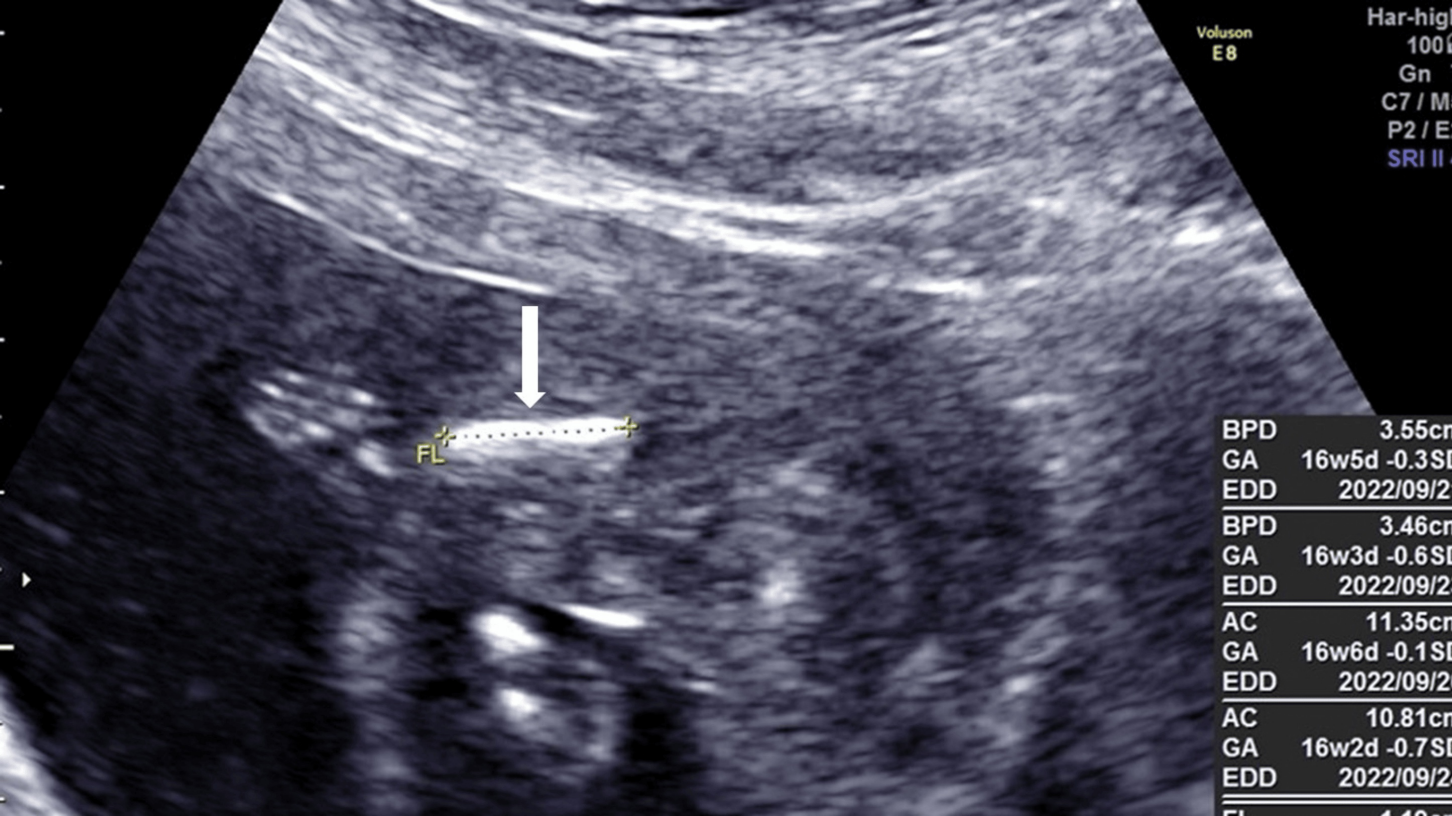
Femur length on ultrasonography of the third pregnancy at 17 weeks of gestation The fetal femur was shortened and curved (white arrow), similar to the second pregnancy.
